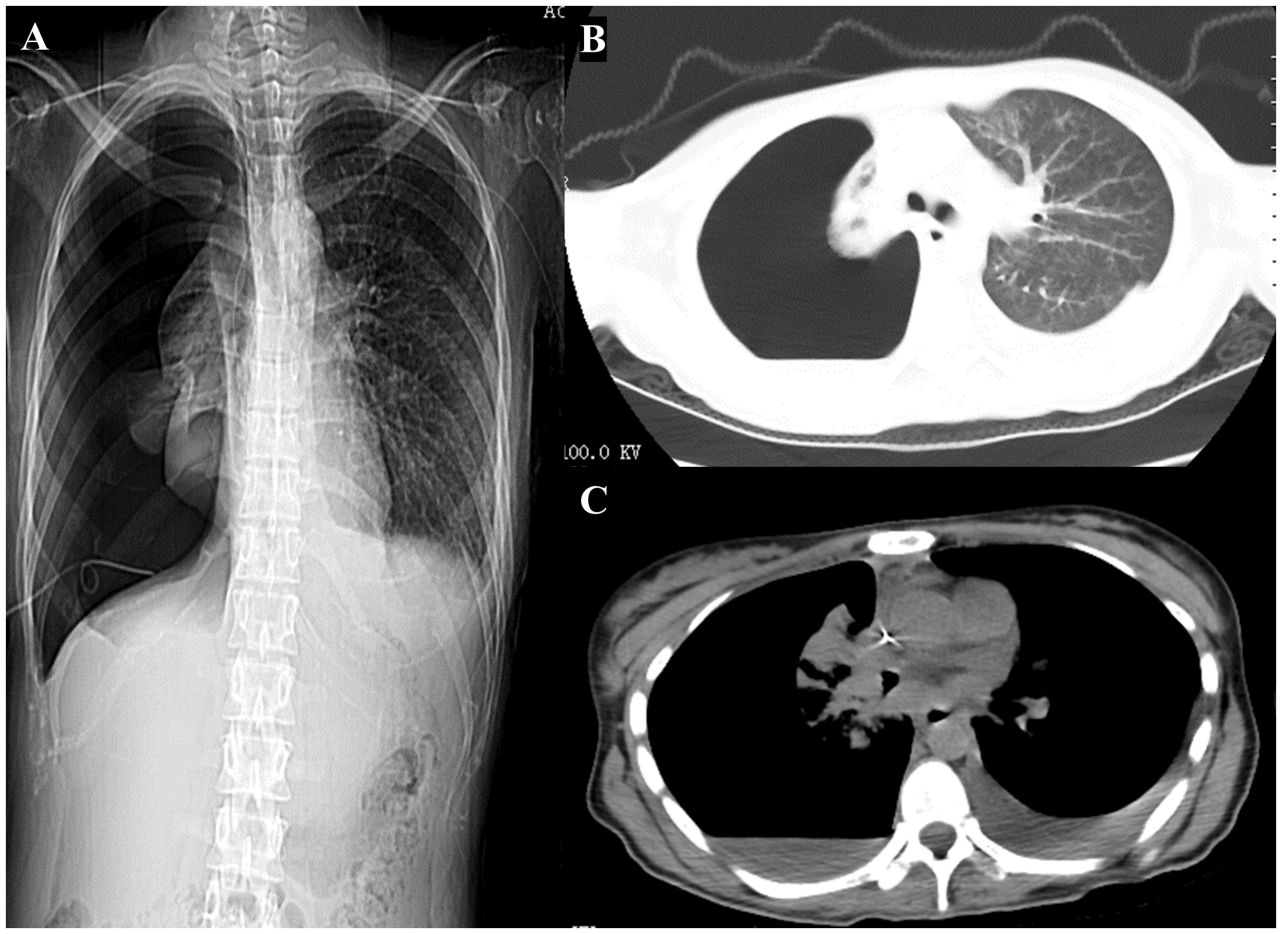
Computed tomography scan of the chest was performed during hers last hospitalization. (A) Chest x-ray imaging. (B, C) Computed tomography scan of the chest showing a right pneumothorax and left pleural effusion.
